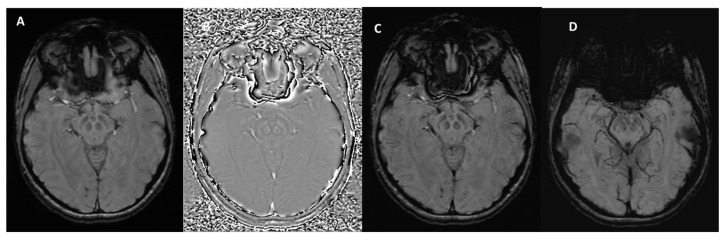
SWI outputs from the Siemens MAGNETOM Aera (Erlangen, Germany) 1.5T MRI scanner at the level of the midbrain. (A) Magnitude Image: displays the intensity of the signal, showing general tissue contrast. (B) Phase Image: highlights variations in magnetic susceptibility, useful for detecting iron deposition and microhemorrhages. (C) SWI-Processed Image: combines magnitude and phase data to enhance the visualization of microvascular structures and pathological features. (D) Minimum Intensity Projection: a multislice projection image that accentuates low-signal structures such as veins and calcifications for improved spatial visualization.
